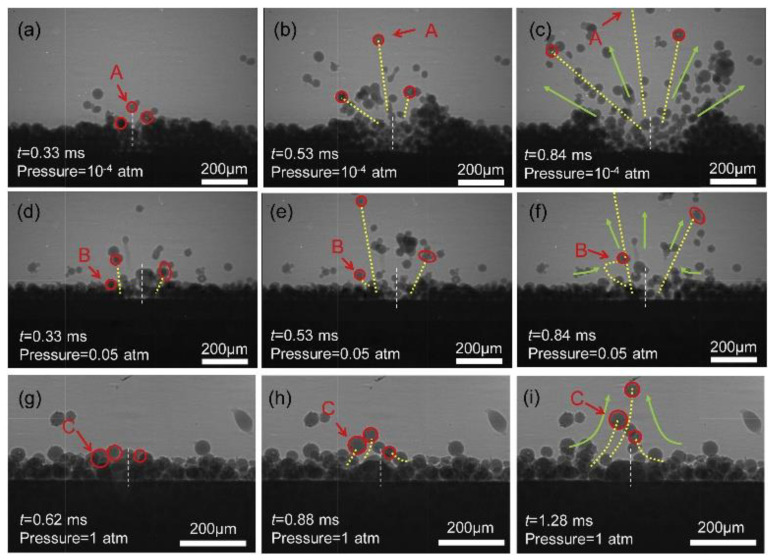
Dynamic X-ray images showing the movement of the powder at different times and pressures [111].
